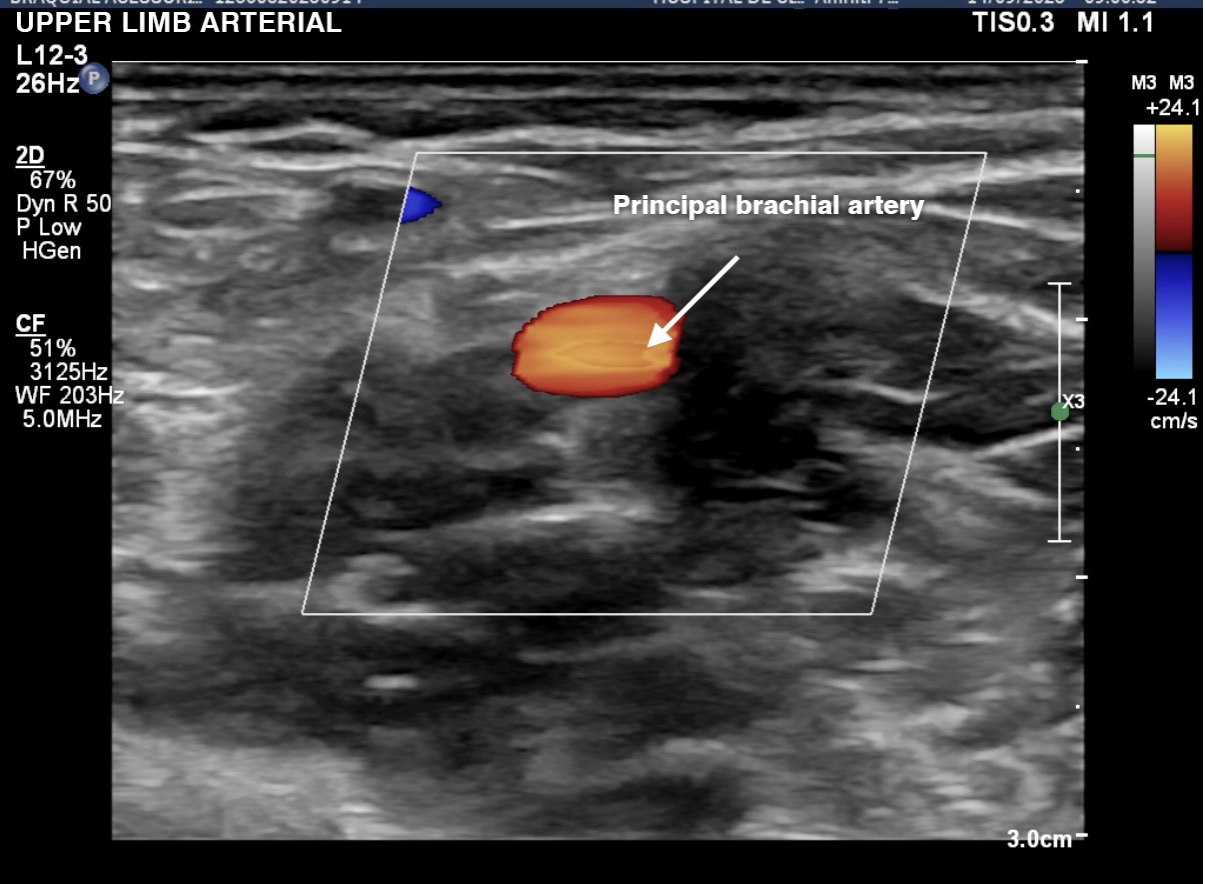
Doppler ultrasound in transverse view showing just the principal brachial artery, indicating that there is no anatomic variant of the artery.
